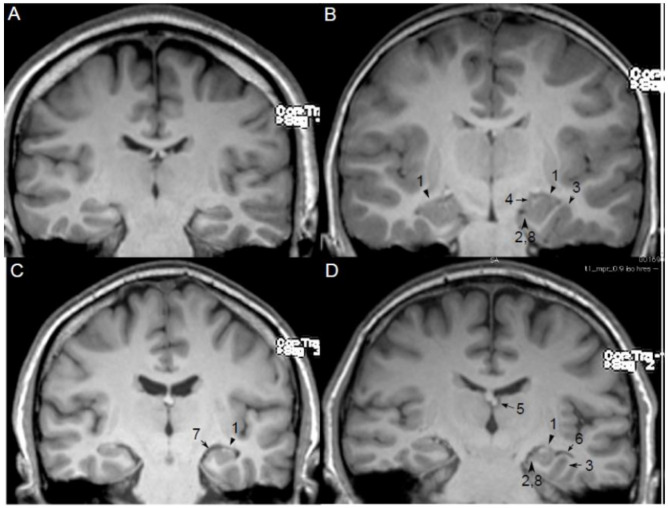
MRI qualitative features of hippocampal malrotation (HIMAL). Figure 1 Legend: (A) Normal bilateral hippocampus, (B) Bilateral HIMAL, (C,D) Unilateral HIMAL on left side. 1 Round or pyramidal shape instead of ovoid shape, 2 medial position of hippocampus on hippocampal sulcus, 3 excessively deep or “verticalized” collateral sulcus, 4 medial located fimbria, 5 small or displaced fornix, 6 enlarged temporal horn, and empty choroid fissure, 7 thickened subiculum, 8 reduced upper horizontal portions of the parahippocampal gyrus.
